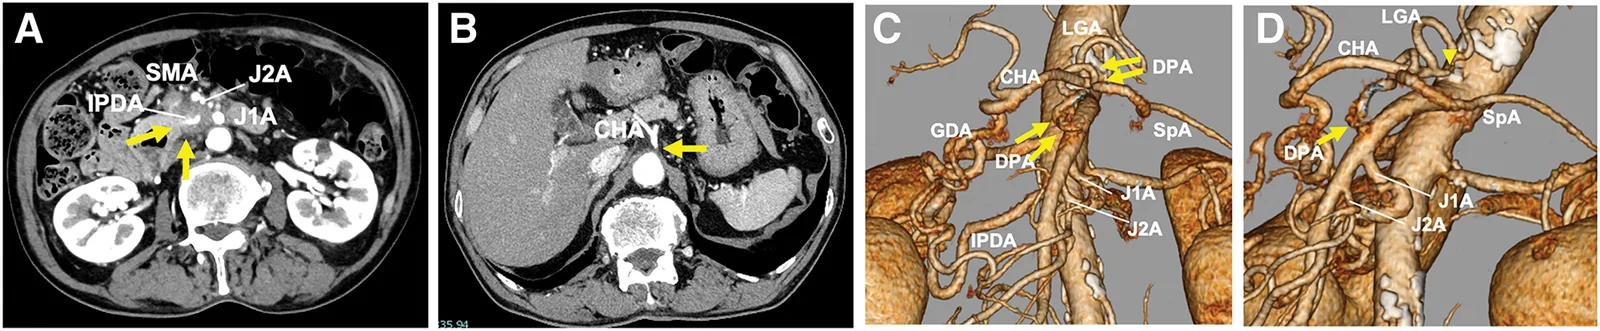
(A) Contrast-enhanced abdominal CT revealed a 31-mm tumor in the pancreatic head (yellow arrow) abutting the SMA and involving the J1A and J2A. (B) CA occlusion due to MAL compression (yellow arrow). (C) 3D CT angiography images demonstrating the vascular anatomy from a frontal view. The DPA (yellow arrow) originates from the anterior surface of the SMA cranial to the tumor (green), with no evidence of tumor involvement. (D) 3D CT angiography images demonstrating the vascular anatomy from an oblique view. CA occlusion due to MAL compression (yellow arrowhead). The origin of the DPA (yellow arrow) is distinct from the origin of the J1A. In Fig. 1C and 1D, the J1A collateral artery was omitted to more clearly demonstrate the course of the DPA. CA, celiac artery; DPA, dorsal pancreatic artery; J1A, first jejunal artery; J2A, second jejunal artery; MAL, median arcuate ligament; SMA, superior mesenteric artery
